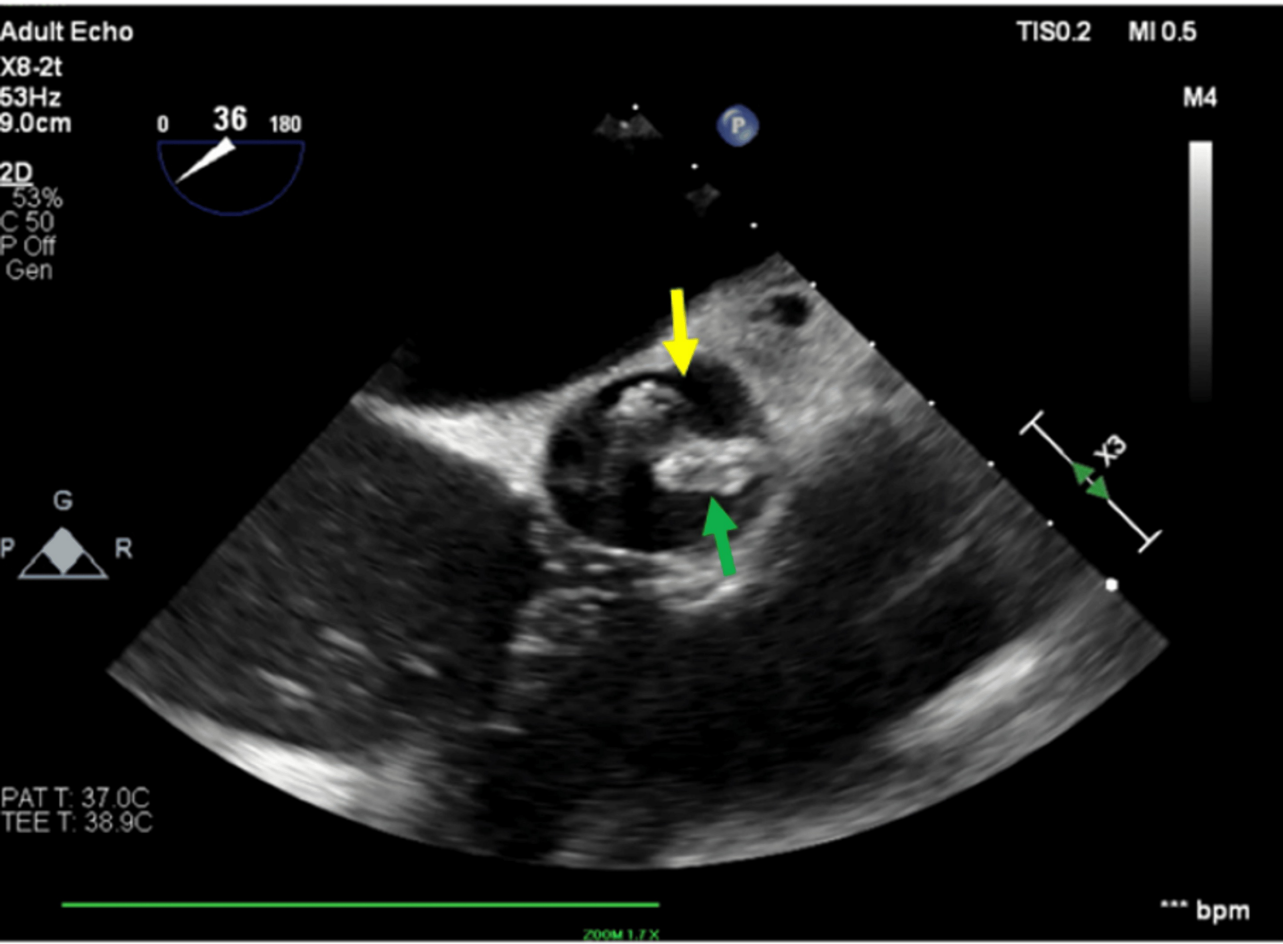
Transesophageal echocardiogram (TEE) short-axis view findings TEE showing a vegetation on the right coronary cusp (green arrow) and a smaller vegetation on the left coronary cusp. The yellow arrow shows the left main coronary artery.
